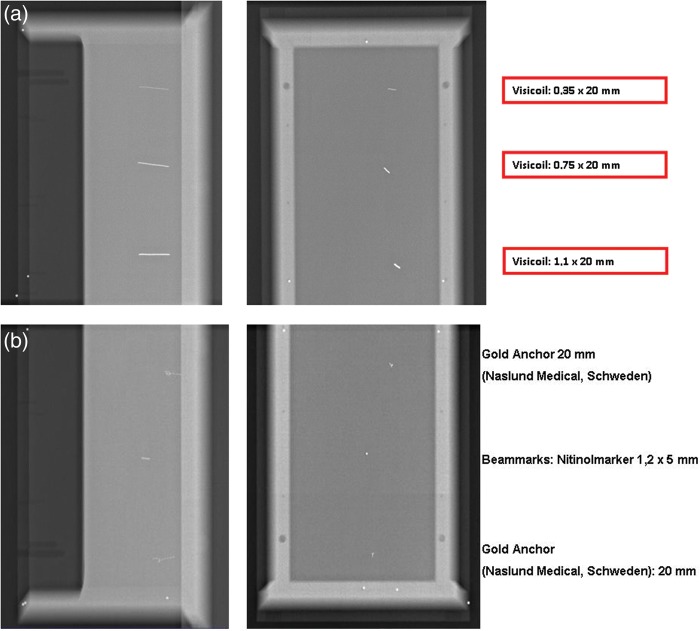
CT scan of different markers in gelatin solution. Front section (a) containing three different sized Visicoil markers (sizes from top to bottom: 0.35 x 20 mm, 0.75 x 20 mm, 1.1 x 20 mm). Back section (b) containing Gold Anchor marker (0.27 x 20 mm) in first and last position and Beammarks (1.2 x 5 mm).
